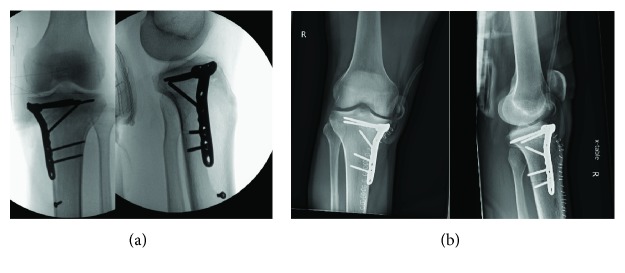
(a) Postoperative radiographs of left knee status post excision, grafting, and open reduction internal fixation left proximal tibia. (b) Postoperative radiographs of right knee status post excision, grafting, and open reduction internal fixation right proximal tibia.
